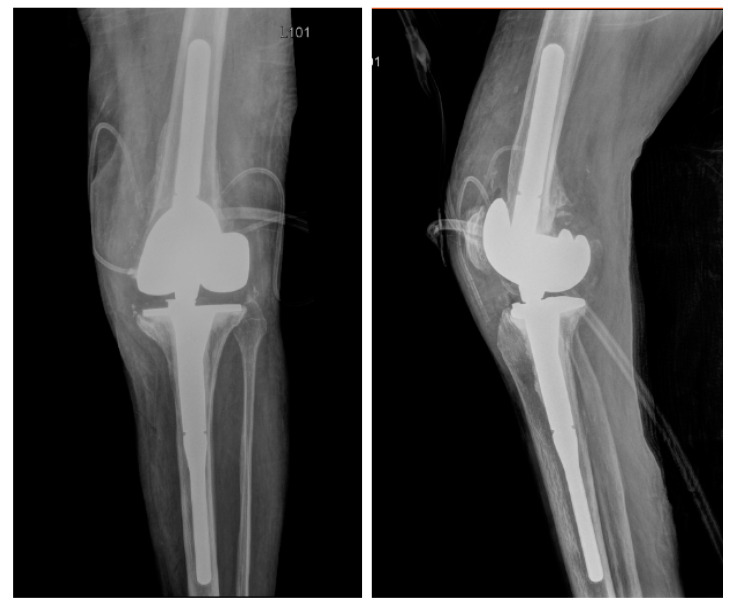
Post-operative anterior–posterior and lateral X-ray showing well fixed long stem femoral and tibial arthroplasty components. No hardware complication is present but generalized osteopenia and expected postoperative changes with intra-articular emphysema. Surgical drain is also noted.
